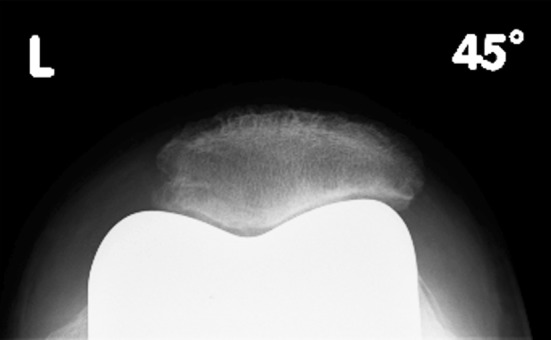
Skyline radiograph obtained 3 years following TKA demonstrating signs of biological remodelling (‘stress contouring’) of the retro-patellar surface
